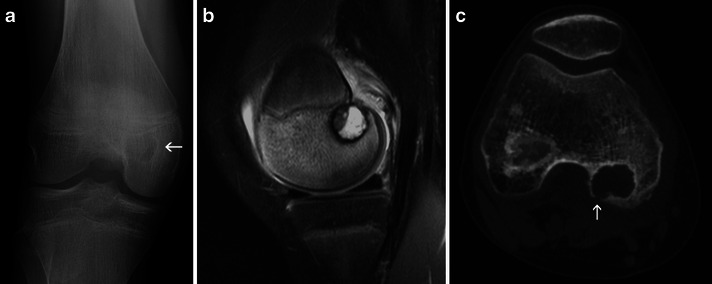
Typical chondroblastoma of the distal femoral epiphysis: a Frontal X-ray of the right knee: well-defined round osteolytic lesion of the distal femoral epiphysis (*arrow*). b Sagittal fat-saturated proton density MRI: the lesion is well defined, mostly hyperintense, surrounded by bone oedema and an inflammatory reaction of the adjoining soft tissues. c CT of the right knee: the cortex is interrupted on the posterior border of the lesion (*arrow*), but no periosteal reaction is identified
